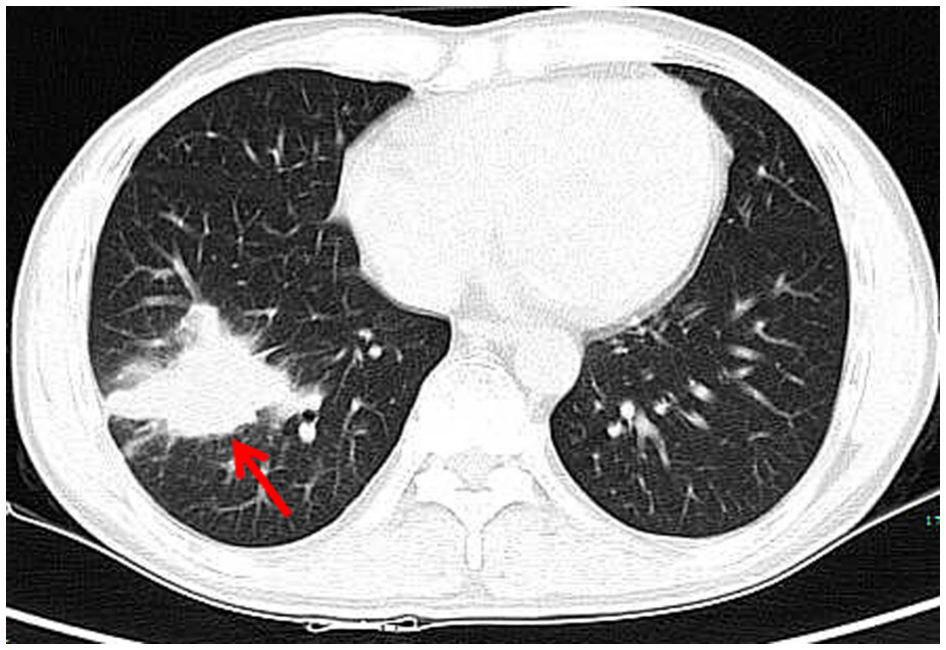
Chest CT showed that the right lower lung mass had not shrunk after anti-infective treatment (red arrow).
